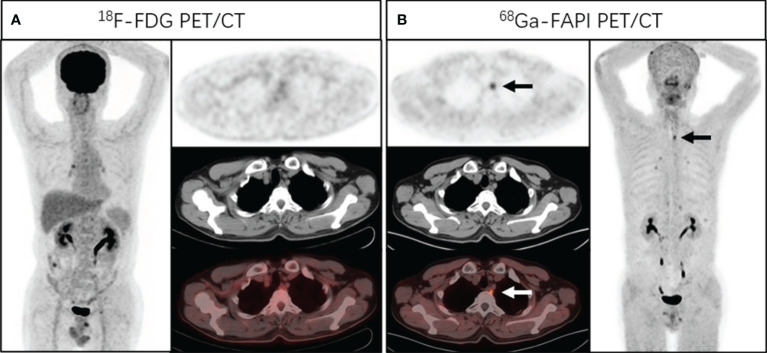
A 58-year-old man presented with a complaint of progressive dysphagia and back pain for 2 months. Endoscopic biopsy demonstrated a well-differentiated squamous cell carcinoma of the upper esophagus. Chest CT and [^18^F]FDG PET/CT (A) showed no abnormal density and activity in esophagus. [^68^Ga]Ga-DOTA-FAPI-04 PET/CT (B) revealed a focus of intensive uptake in the upper esophageal cancer (arrows, SUVmax of 3.4); no other foci of [^68^Ga]Ga-DOTA-FAPI-04 uptake associated with esophageal cancer were seen. Subsequently, the patient received radical radiotherapy plus chemotherapy.
